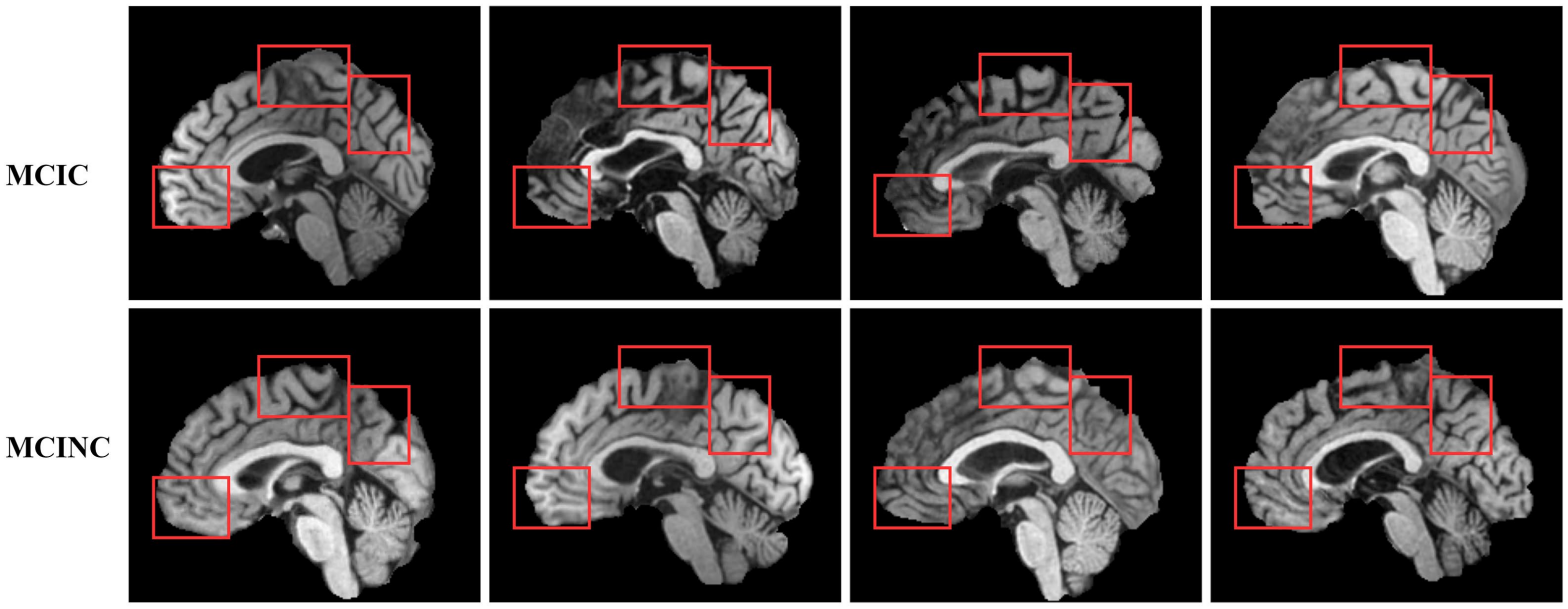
Example of MCIC and MCINC MRI scans with region of interest (ROI).
